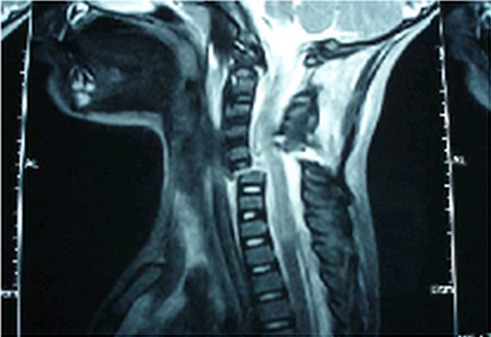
Medullary full section on a sagittal section of MRI of the cervical spine
